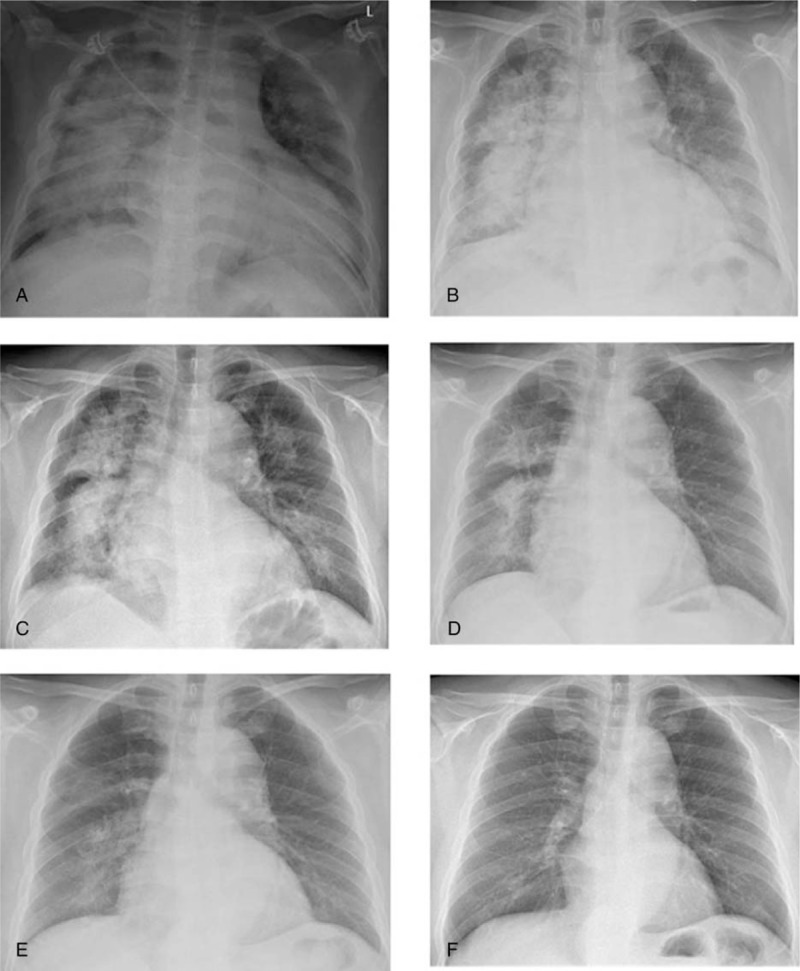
The chest X-ray manifestations of this patient. Since the first visit, the change of the patient's chest X-ray manifestations on the (A) 1st day, (B) 3rd day, (C) 1st week, (D) 2nd week, (E) 4th week, and (F) 8th week.
